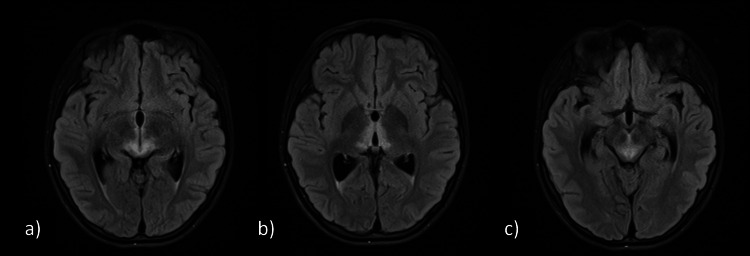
Flair axial MRI brain MRI: Magnetic resonance imaging Bilateral nearly symmetric areas of signal intensity in the posterior (a) and medial (b) thalami as well as the periaqueductal region of the midbrain (c). No significant interval change compared to the previous scan done on 4 May 2025. No abnormal signal identified in the basal ganglia, cerebral or cerebellar white matter, or optic pathways. The orbits appear unremarkable. No signs of optic nerve signal abnormality or atrophy. No hydrocephalus or midline shift. No abnormal intracranial enhancement.
